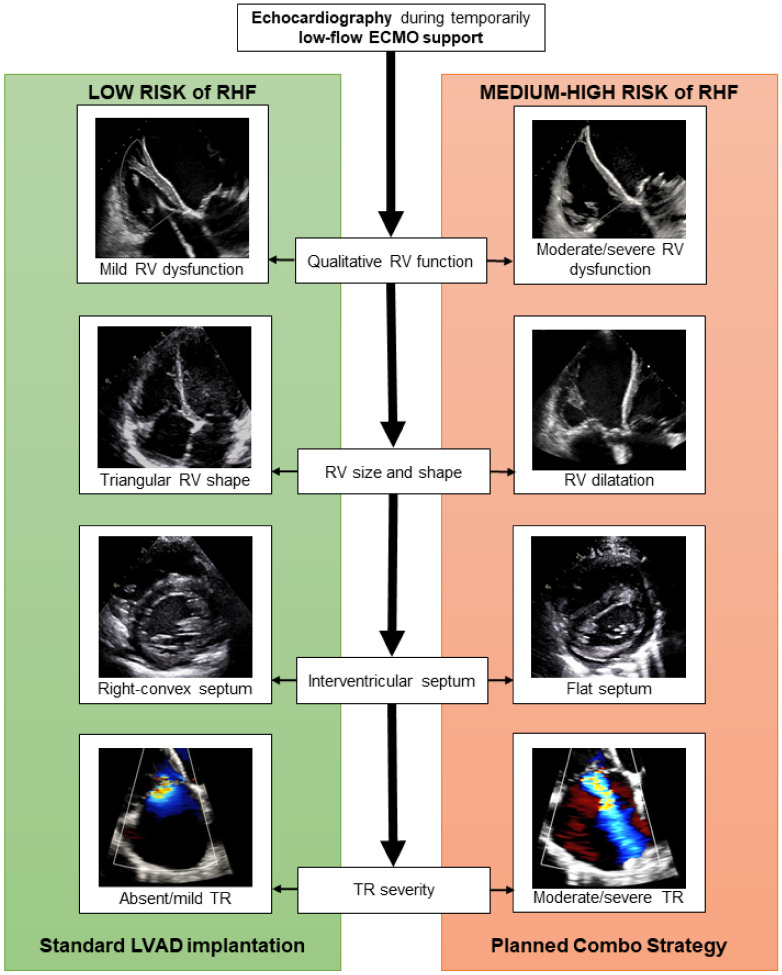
Echocardiographic parameters of RV performance that guide d-LVAD implantation strategy in ECMO patients. RV: right ventricle; TR: tricuspid regurgitation.
